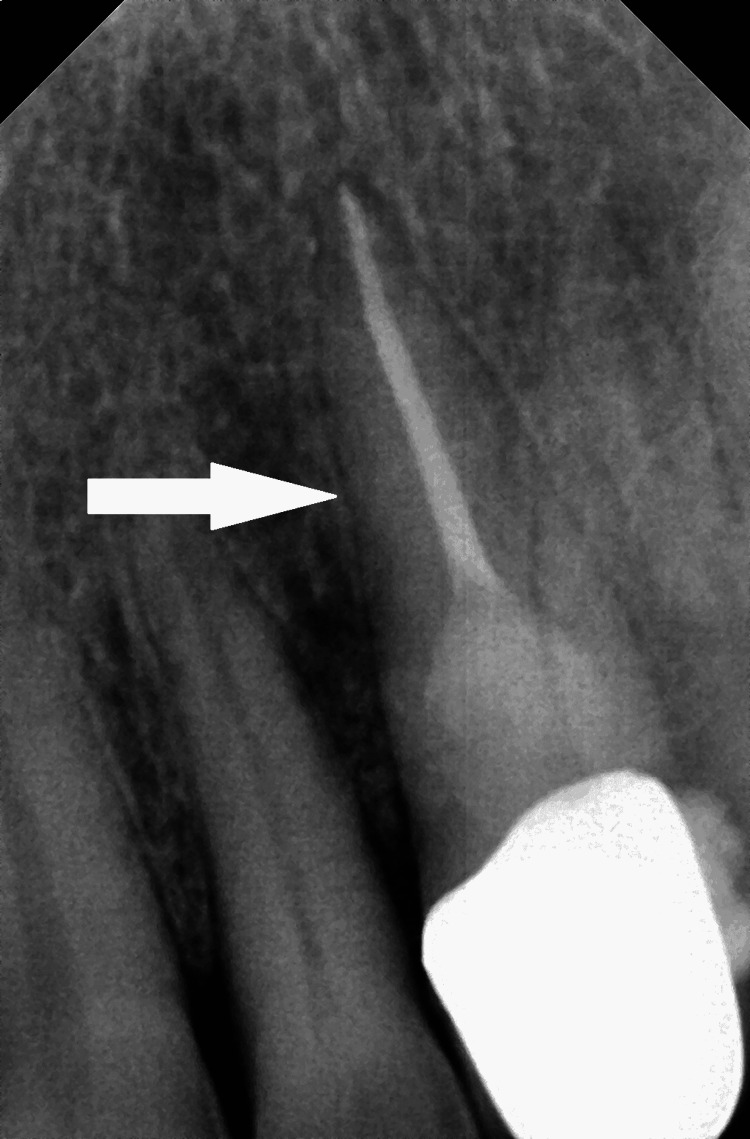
12 months follow up radiograph
